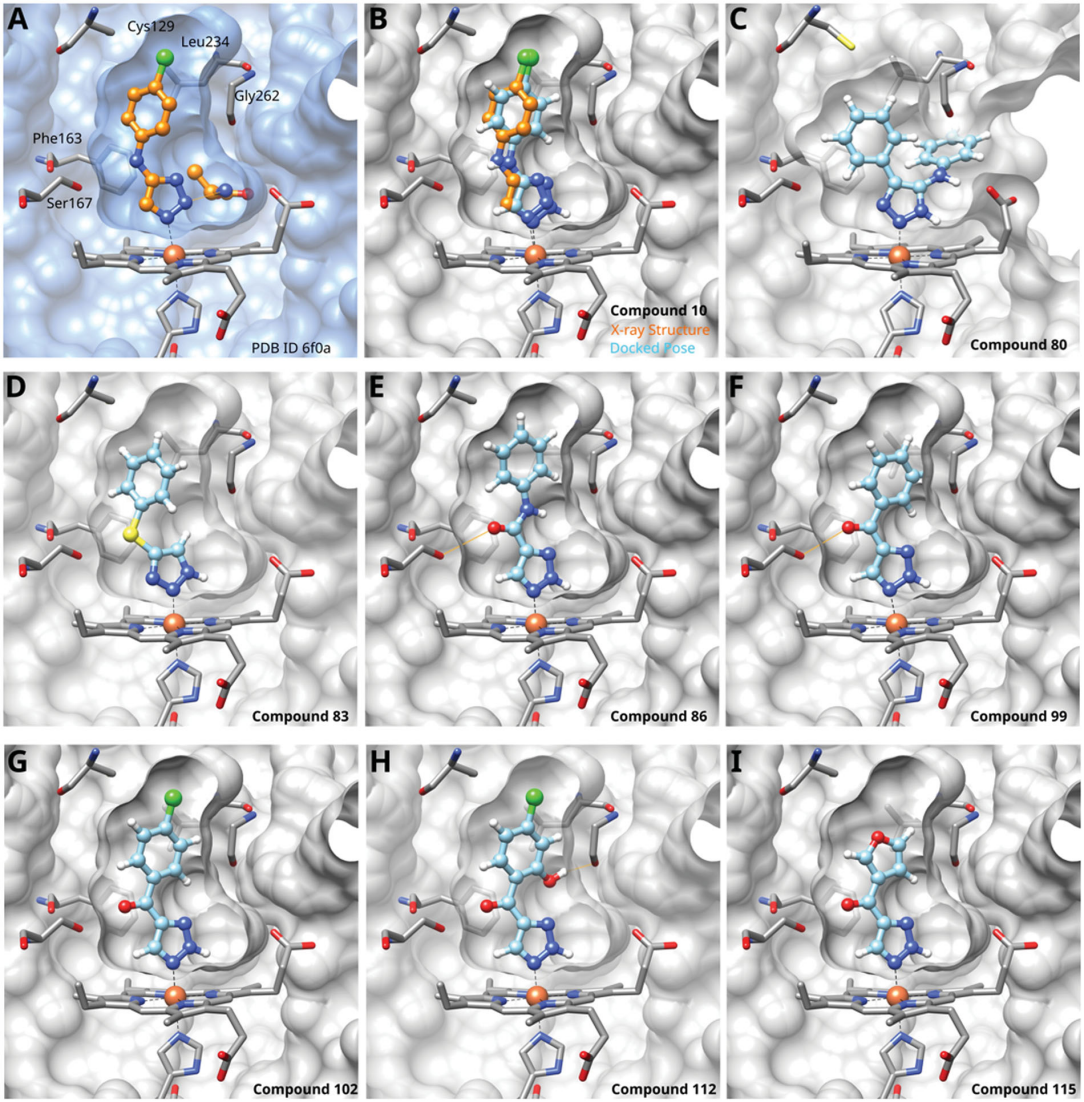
Tethered 1,2,3-triazoles. (A) X-ray structure of compound 10 (PDB ID 6f0a)^60^. (B) Superposition of X-ray structure and of docking pose of 10. Docking poses of (C) compound 80; (D) compound 83; (E) compound 86; (F) compound 99; (G) compound 102; (H) compound 112; (I) compound 115. Hydrogen bonds are shown in orange.
